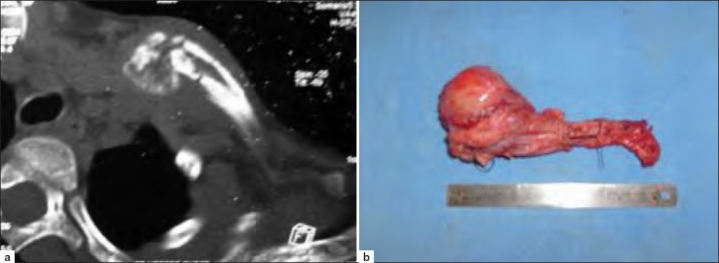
(a) Axial section of noncontrast CT scan showing Ewing’s sarcoma of the right clavicle. (b) Photograph showing the resected right clavicle with tumor *in situ*.
